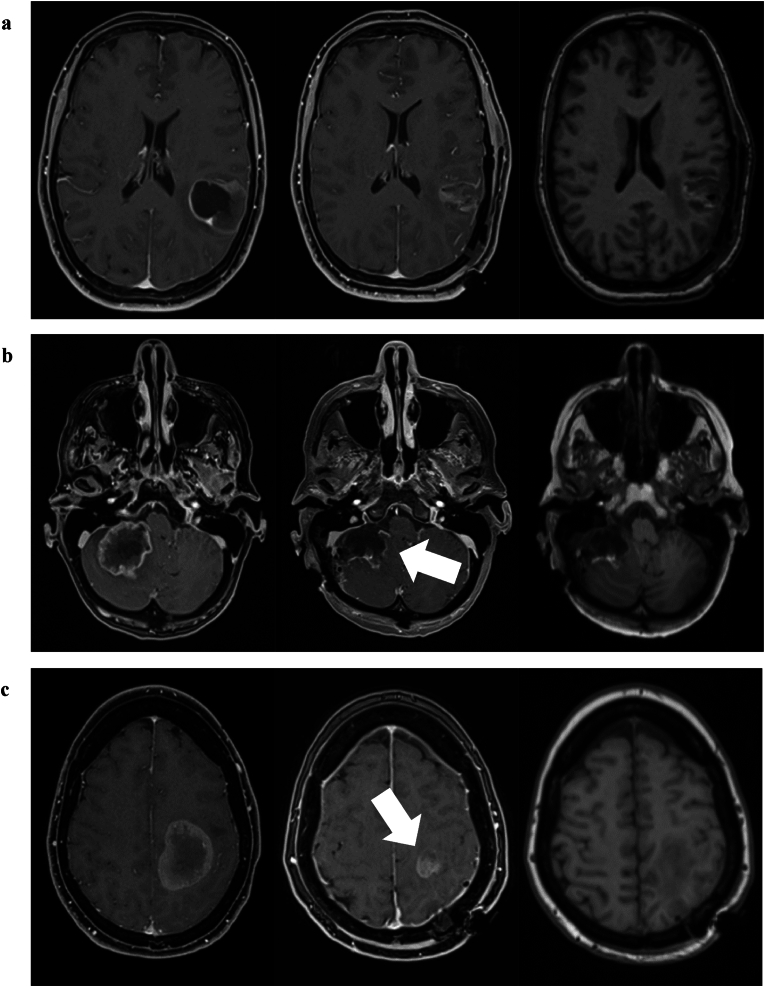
Extent of resection assessed by early postoperative MRI. a 46-year-old female with HER2 positive, ER/PR negative breast cancer. She suffered a secondary generalized focal onset seizure which led to cranial imaging and the diagnosis of a single 40 mm brain metastasis. Surgery was uneventful. No postoperative contrast enhancement, i.e. complete resection. b 57-year-old female with non-small cell lung cancer. She presented with headaches, dizziness, nausea and emesis in a short period of time. Surgery was performed to remove the space-occupying lesion in the posterior fossa which compressed the IV. ventricle and carried a risk for occlusive hydrocephalus. Very thin shell-like layer of contrast enhancement on the medial resection border (white arrow). The neuroradiological review classified this as questionable residual tumor. c 54-years-old female with an adernocarcinoma of the pancreas. The patient had developed mild hemiparesis on the right side. Surgery was performed for cytoreduction but also aiming to prevent further neurological worsening. The patient indeed remained neurologically stable following the surgery. Nodular tumor remnant (white arrow) in the central region left behind during surgery because of (reversible) deterioration of intraoperative MEP recordings.
